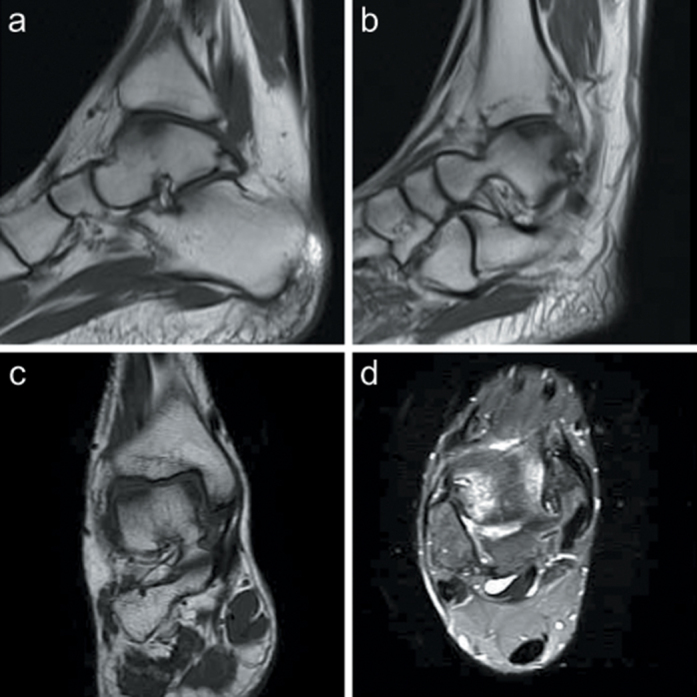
Preoperative magnetic resonance images. (a) Preoperative T1 weighted sagittal magnetic resonance images of OLT on medial talar dome, (b) T1 weighted sagittal magnetic resonance images of OLT on lateral talar dome, (c) T1 weighted coronal magnetic resonance images of OLT on medial and lateral side simultaneously, and (b) T2-weighted axial magnetic resonance images of OLT on medial and lateral talar domes.
